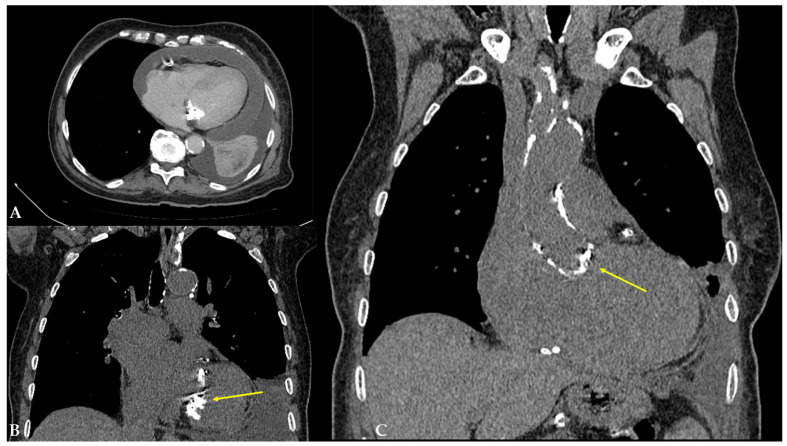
(A) Contrast-enhanced CT of the lung basis displayed moderate pericardial effusion and pleural effusion. (B) Non-contrast-enhanced CT of the thorax revealed an enlarged heart with extensive calcifications of the tricuspid and mitral valves (arrow). (C) Non-contrast-enhanced CT of the thorax demonstrated a highly calcified thoracic aorta wall and aortic valve (arrow).
